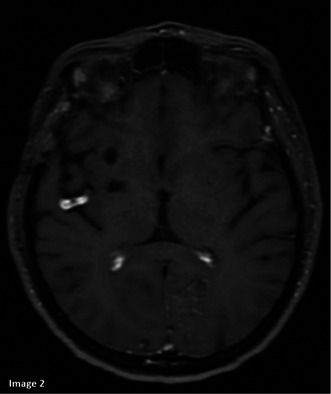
IMAGE 2 Axial T1‐weighted VW MRI after contrast injection showing concentric thickening of one of the aneurysms located in the M2 segment of the right middle cerebral artery, with strong contrast enhancement.
